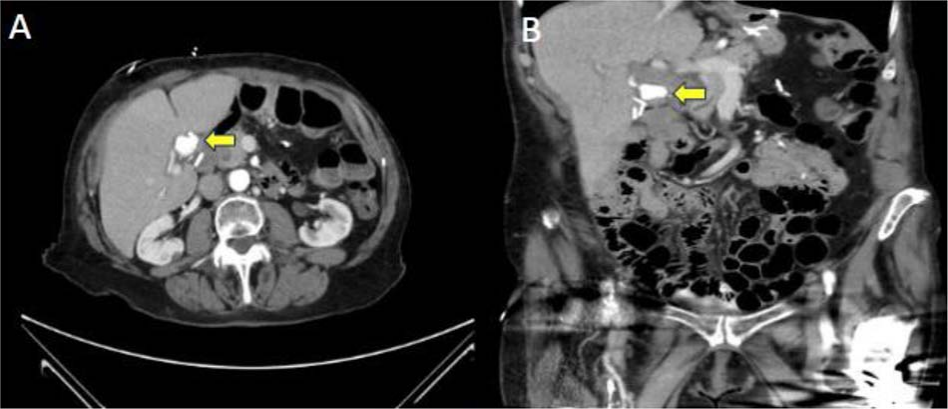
Computed tomography (CT) of the abdomen/pelvis in axial (A) and coronal (B) demonstrating a hyper-enhancing mass in the hepatic hilum (marked by arrow).
